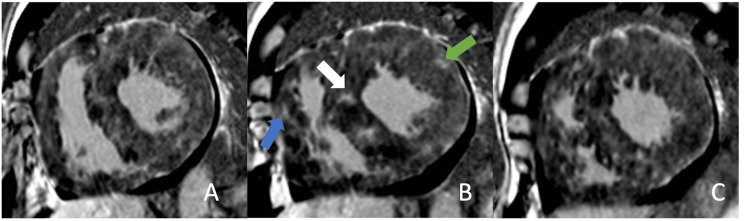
Cardiac MRI Short-axis delayed post-contrast phase-sensitive inversion recovery (PSIR) at the (A) basal, (B) mid-cavity, and (C) apical levels. Extensive biventricular myocardial thickening from the base to apex with foci of patchy, nodular, and hazy abnormal delayed myocardial hyperenhancement is present. These findings are demonstrated in the mid cavity right ventricle (blue arrow), septum (white arrow), and left ventricle (green arrow). Similar findings are also visualized in the basal and apical segments.
